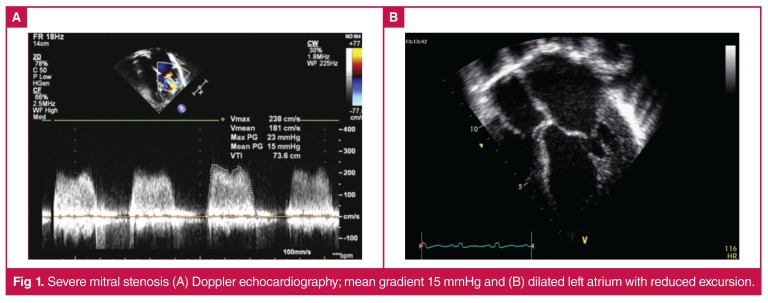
Severe mitral stenosis (A) Doppler echocardiography; mean gradient 15 mmHg and (B) dilated left atrium with reduced excursion.
